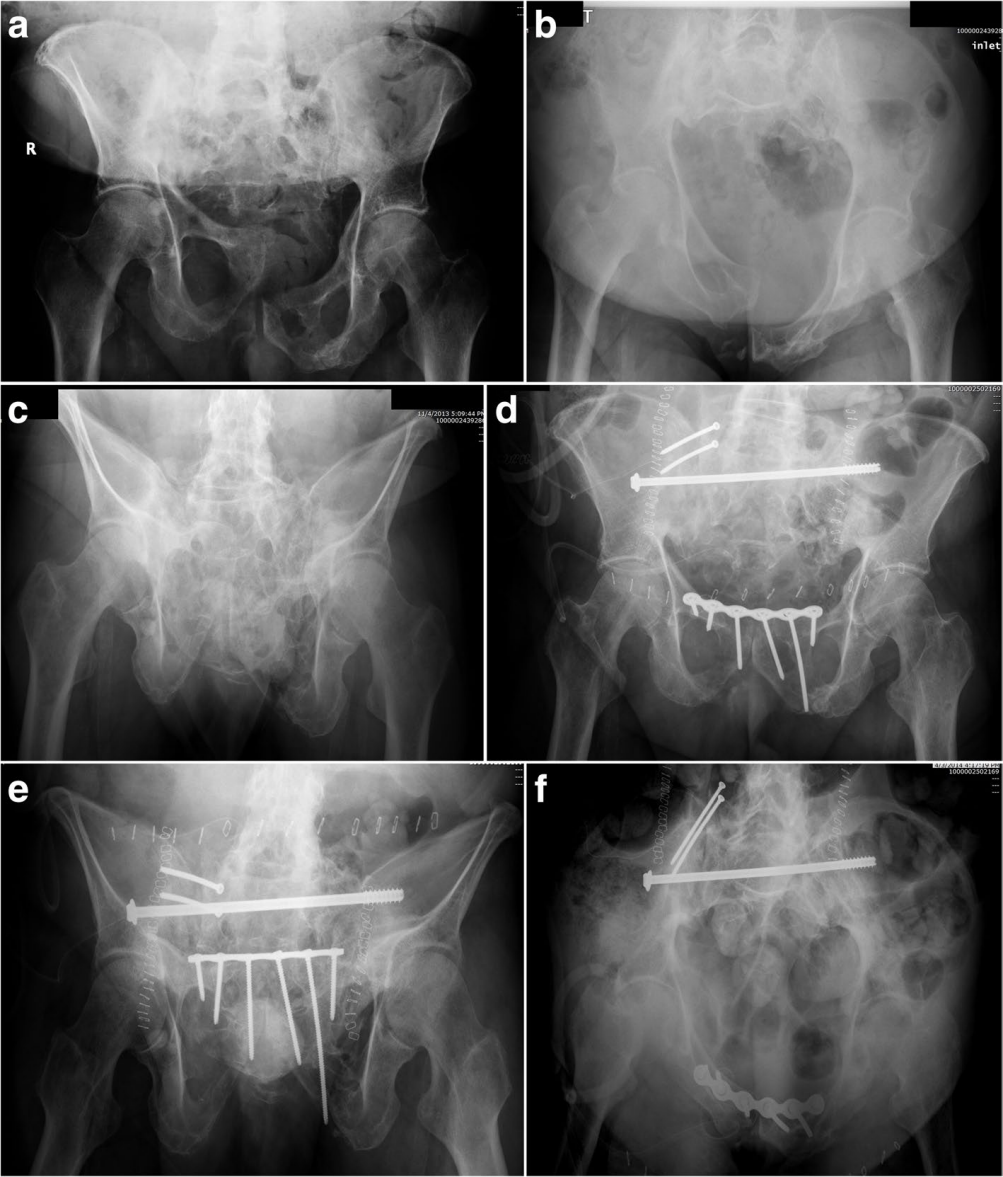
Pre- (A-C) and post-operative (D-F) radiographic images of a pelvic malunion from a right SI fracture-dislocation and pubic symphyseal dislocation. The malunion correction was performed in stages resulting in both anterior and posterior ring fixation
